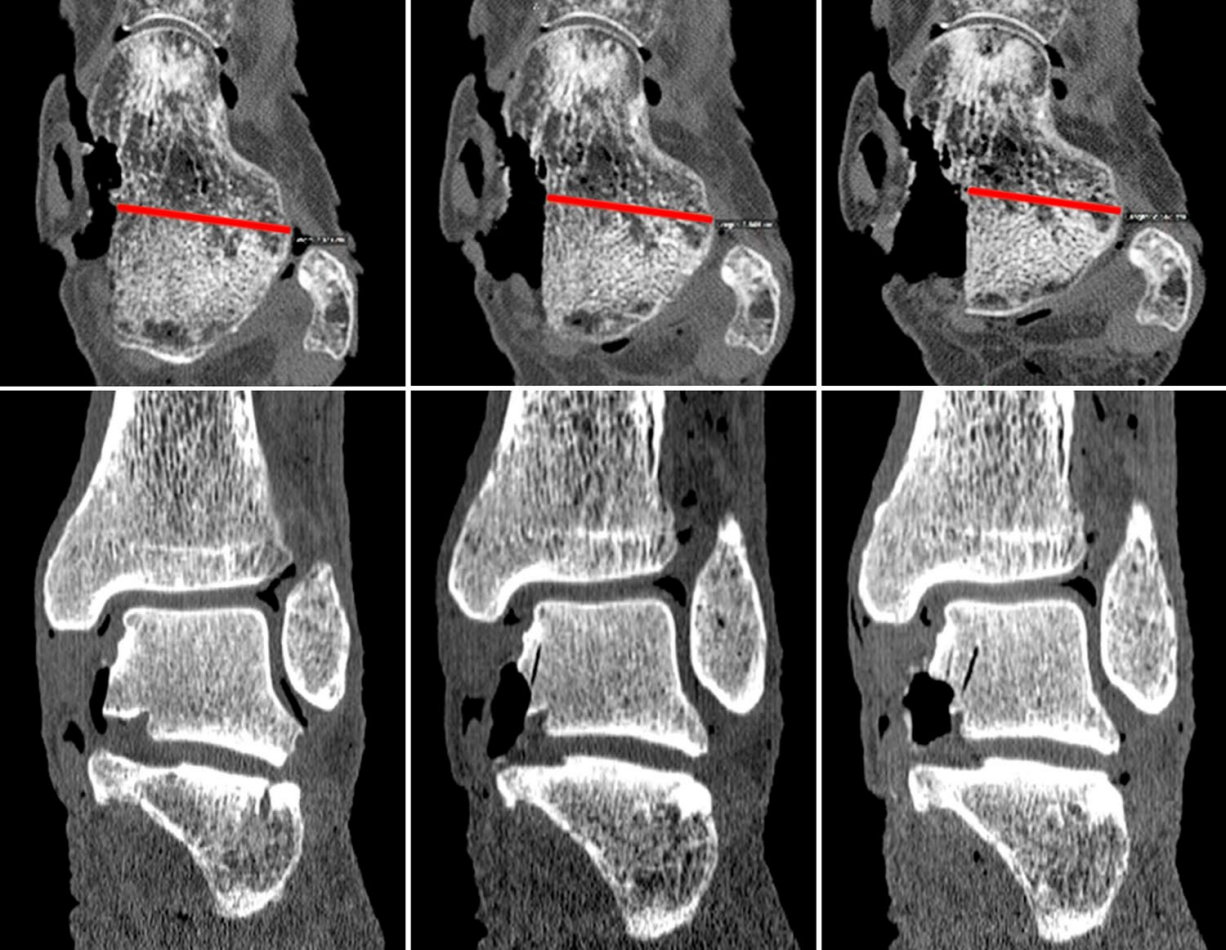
(Top) Axial CT views demonstrating 10, 20, and 30% resections of the medial talar facet and the anteromedial portion of the posterior talar facet; (bottom) coronal CT views demonstrating 10, 20 and 30% resections as described above
